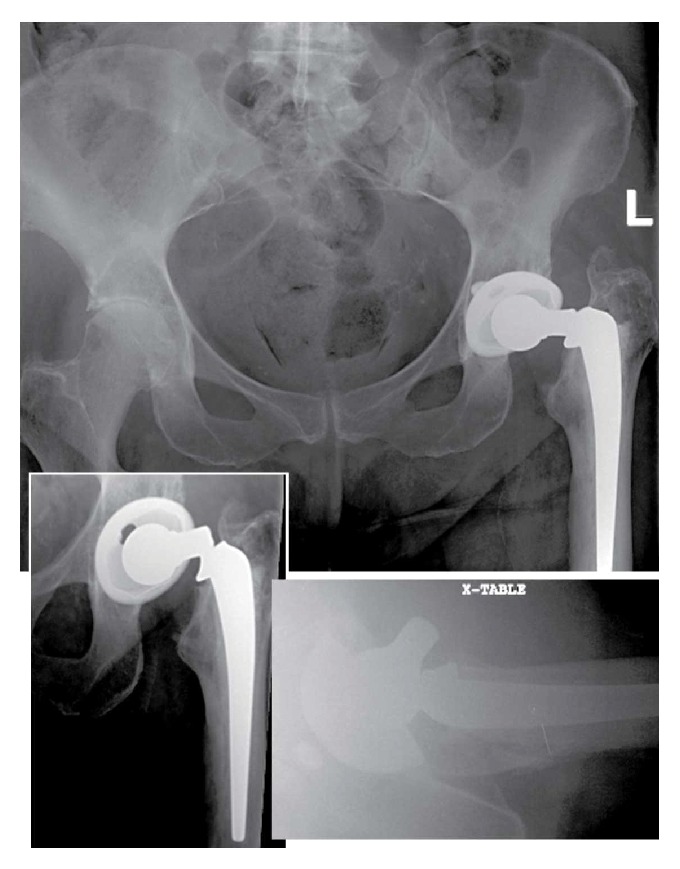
Admission radiographs show femoral component fracture at the neck-shoulder junction at 12 years after implantation.
